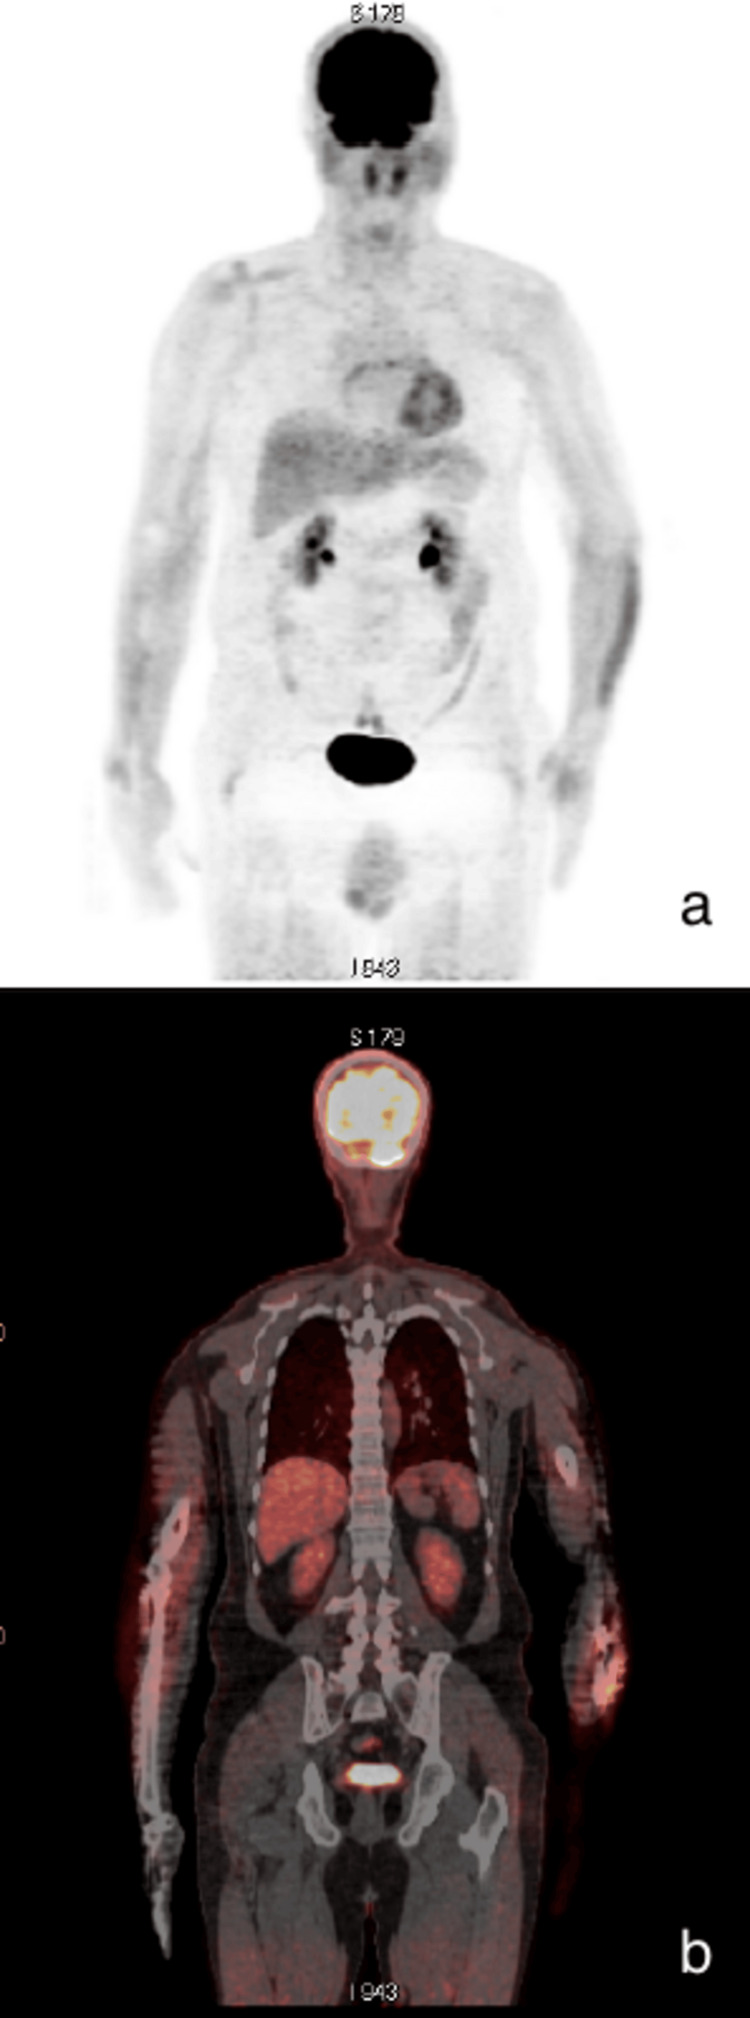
Maximum intensity projection (a) and coronal 18F-fluorodeoxyglucose positron emission tomography/computed tomography fusion (b) showing a total regression of the hypermetabolic lesions.
